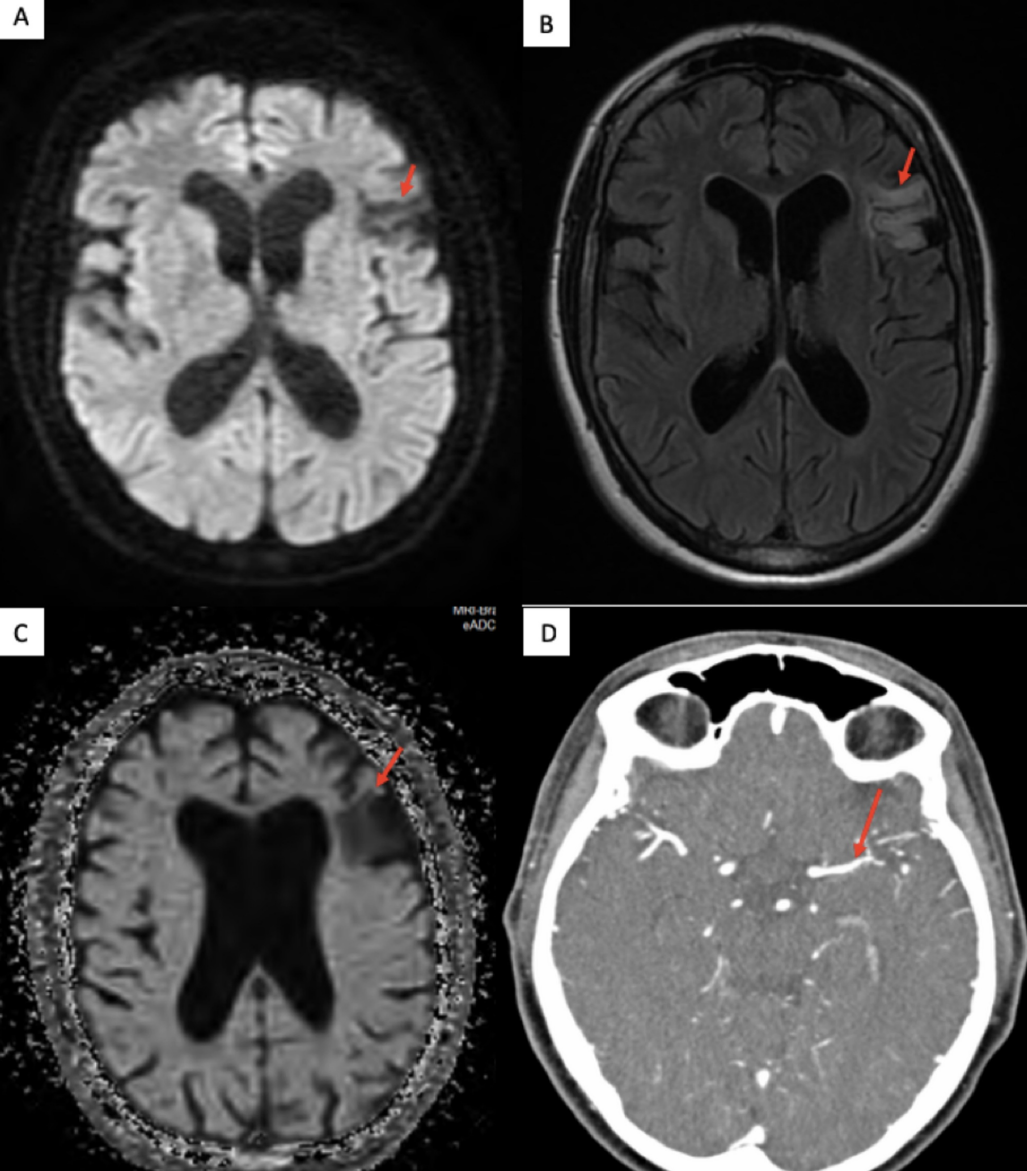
MRI of the brain showing (A) a hypo-intense signal in the left frontal lobe on diffusion-weighted imaging sequence, (B) a hyper-intense signal in the left frontal lobe on T2 flair sequence, (C) a hypo-intense signal on apparent diffusion coefficient sequence, and (D) a mild narrowing left MCA on CT angiography. Abbreviations: MRI, magnetic resonance imaging; MCA, middle cerebral artery; CT, computed tomography
